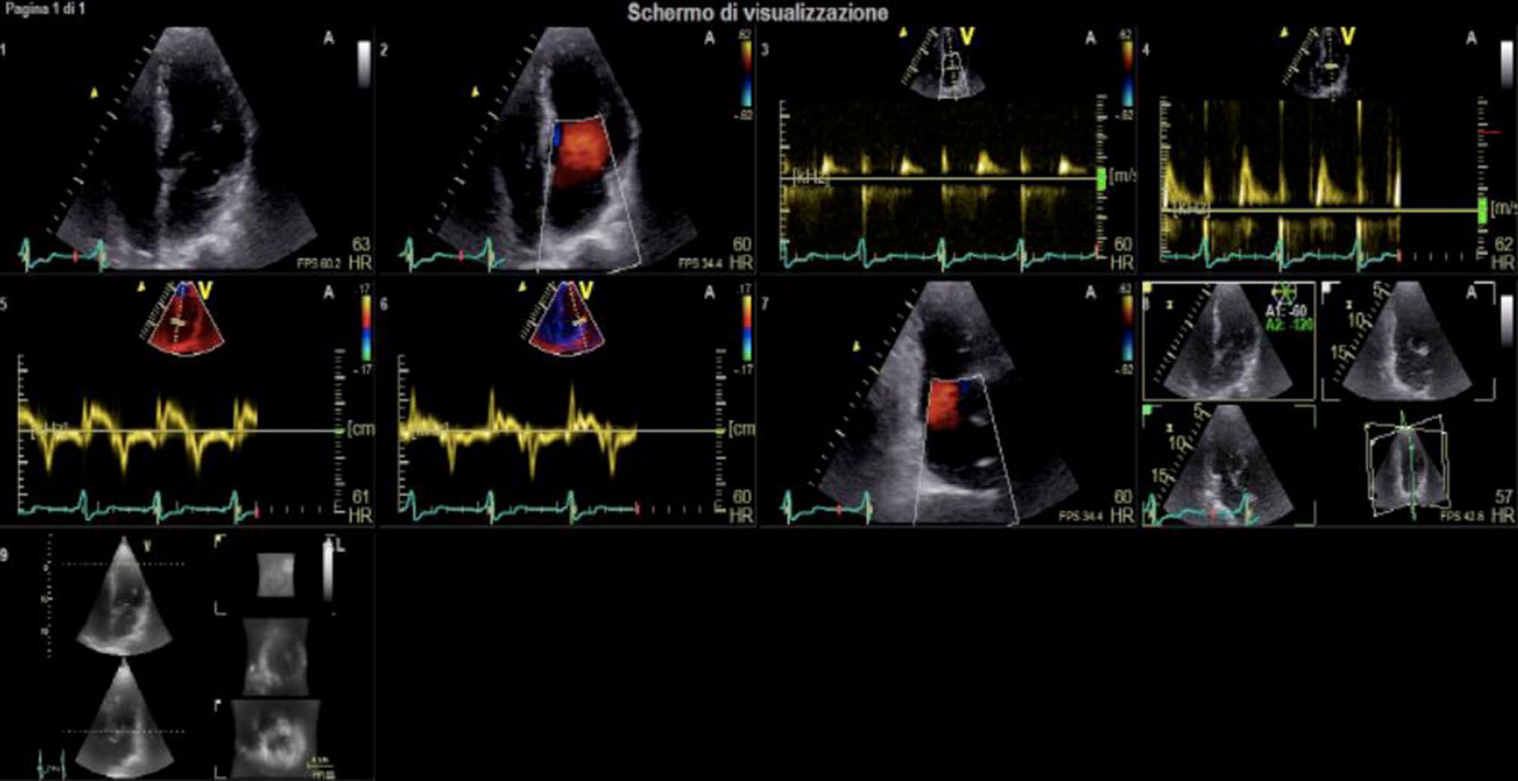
Dataset of echocardiographic acquisitions. Panel of different echocardiographic acquisitions with 2D and 3D probe
